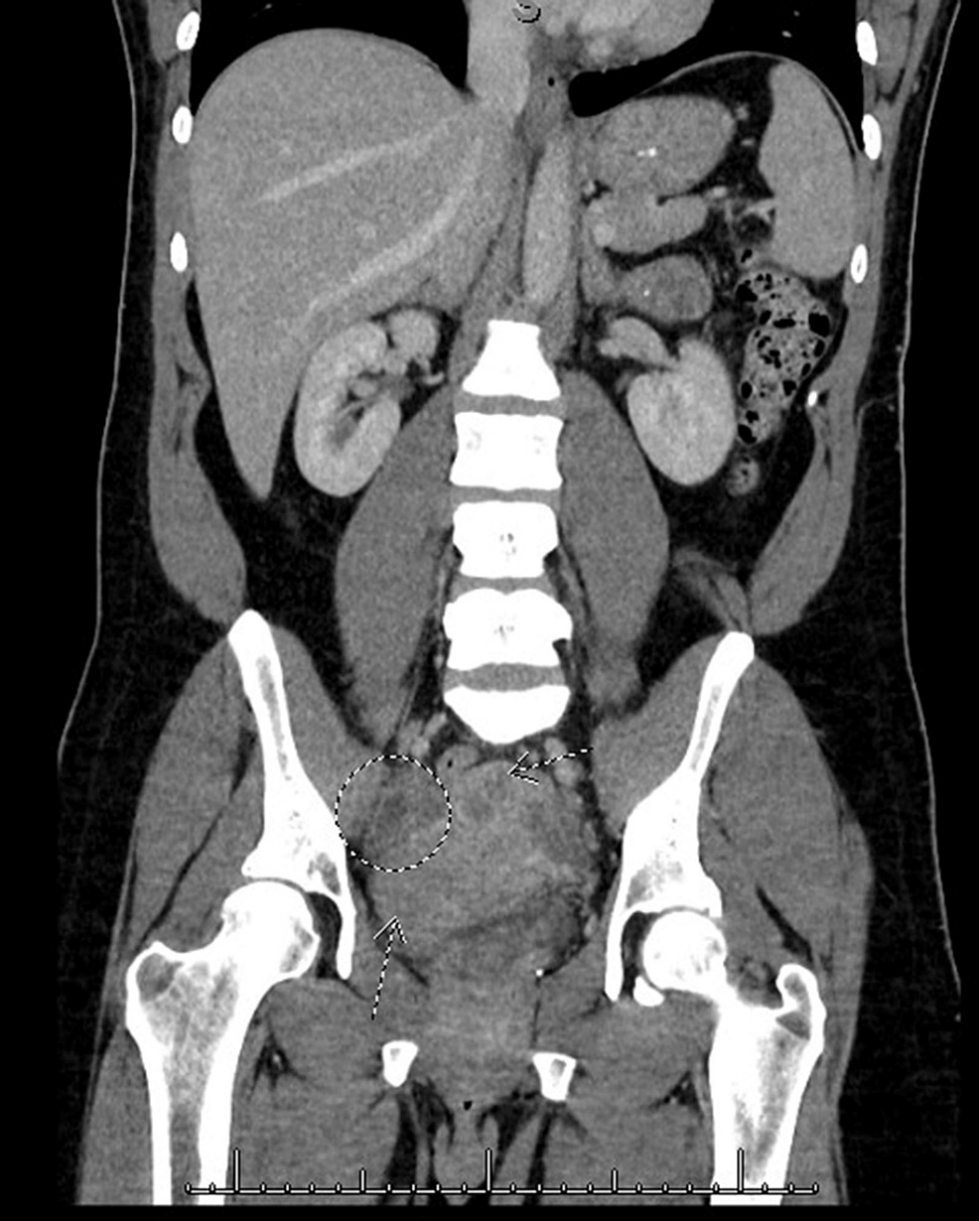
Computed tomography scan of the abdomen Computed tomography of the abdomen and pelvis demonstrating the right ovary with cystic changes (circle) and uterine fibroids (arrows).
